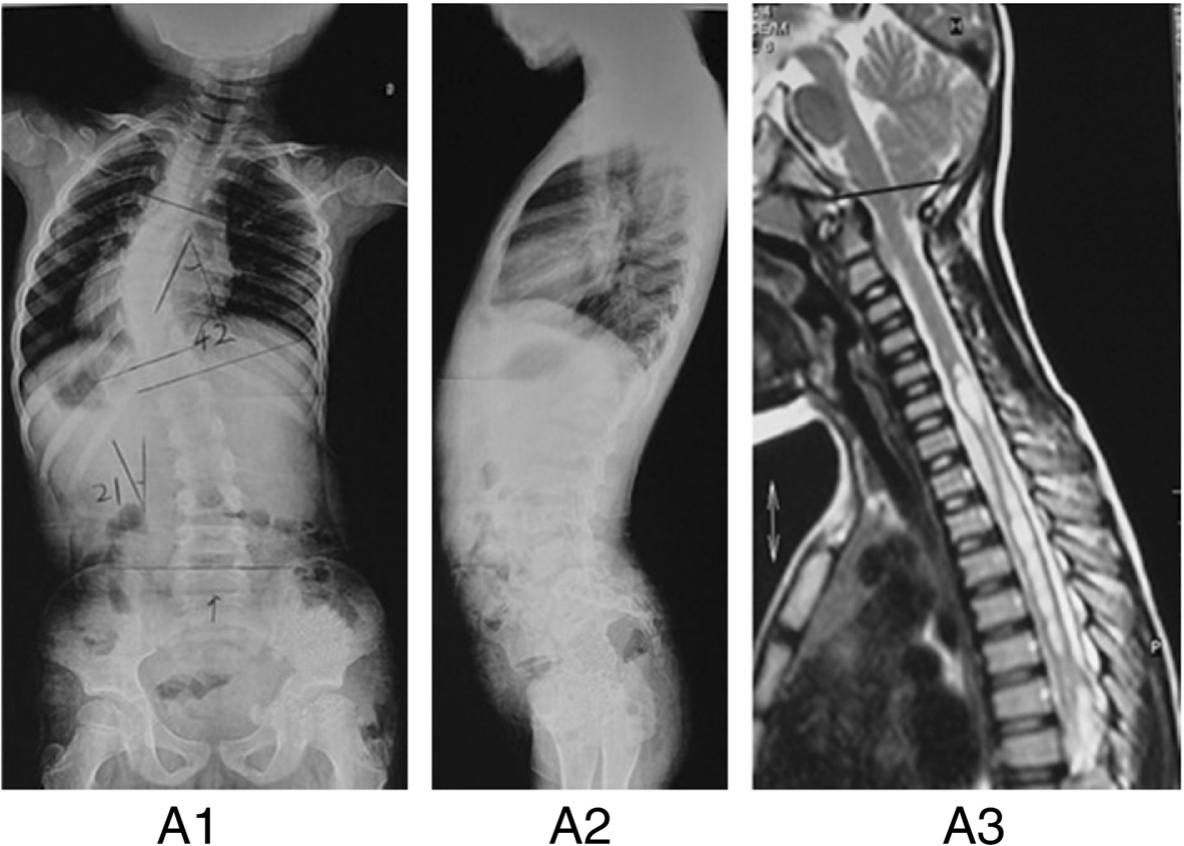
A 8-year-old boy diagnosed as presumed JIS with T5-T11 left-sided thoracic curve of 42° at first visit (A1-A2). He was screened by a full spinal MRI scan which demonstrated an Arnold-Chiari Type-I malformation combined with a moniliform syrinx extending from C5 –T5 seen on the T2 sagittal (A3). Then he underwent PFD (i.e. posterior fossa decompression)
